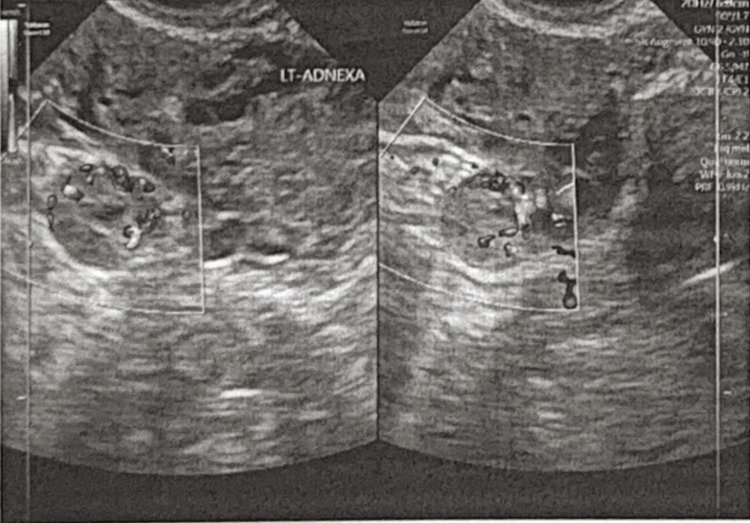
Transvaginal ultrasound showing the left adnexal mass
